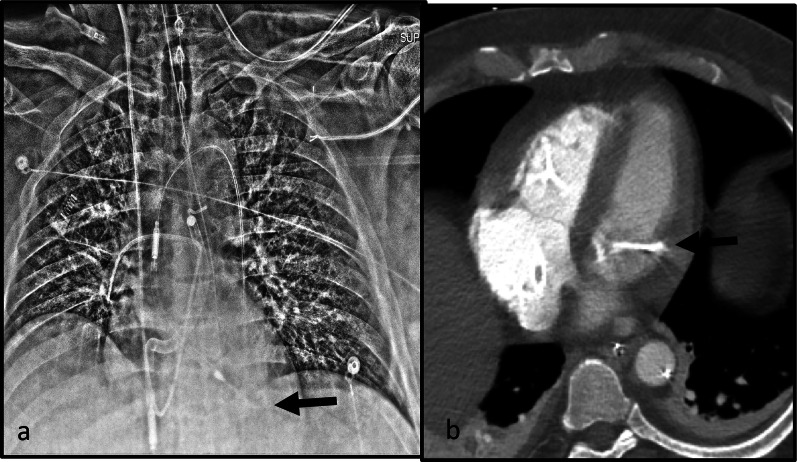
Malposition of Impella device. AP Chest radiograph with CLAHE filter (a) demonstrates a left ventricle Impella device with its tip projecting over the left ventricle (black arrow). Axial contrast enhanced CT (b) demonstrating the pigtail portion of the Impella device is entangled within the papillary muscle apparatus (black arrow). This finding is not detected on radiograph but can be identified on a CT. (CLAHE—contrast limited adaptive histogram equalization)
